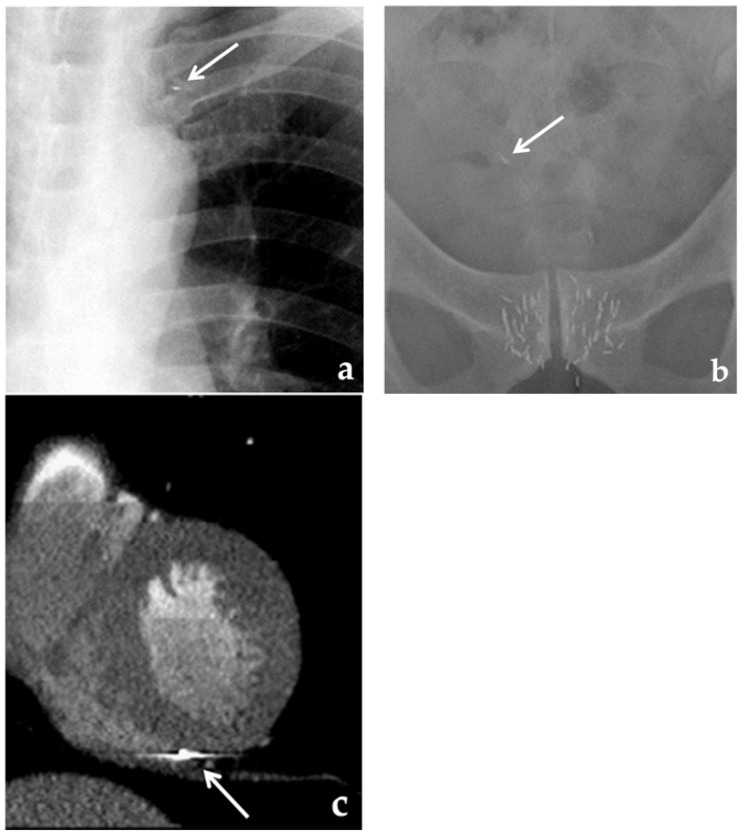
Seed migration after brachytherapy. A follow-up orthogonal chest radiograph showed that a seed had migrated to the left upper lung (solid arrow) (a). A follow-up posteroanterior pelvic radiograph showed that a seed had migrated to the intra pelvic area (solid arrow) (b). Contrast-enhanced CT showed that the seed had migrated to the right ventricle (solid arrow) (c).
